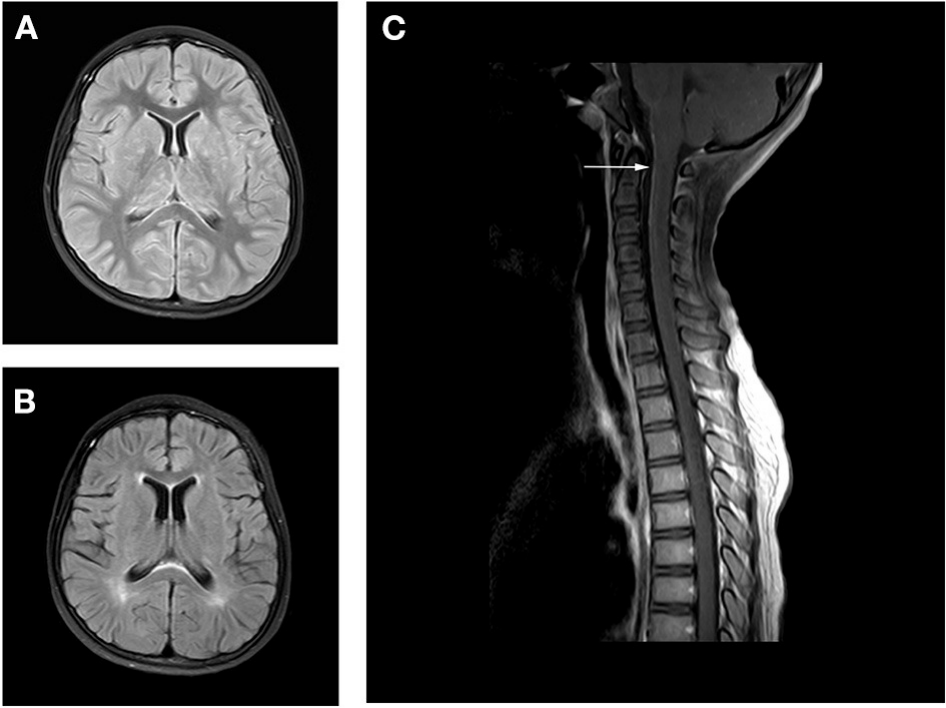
Axial fluid attenuation inversion recovery (FLAIR) MRI brain (A) upon admission and (B) 2 months later. Image (A) showed T2-hyperintense lesions in in bilateral thalamus and basal ganglia. Follow-up image (B) showed improved T2 lesions. MRI of the spine (C) 10 days after admission showed leptomeningeal enhancement in the cervical cord.
